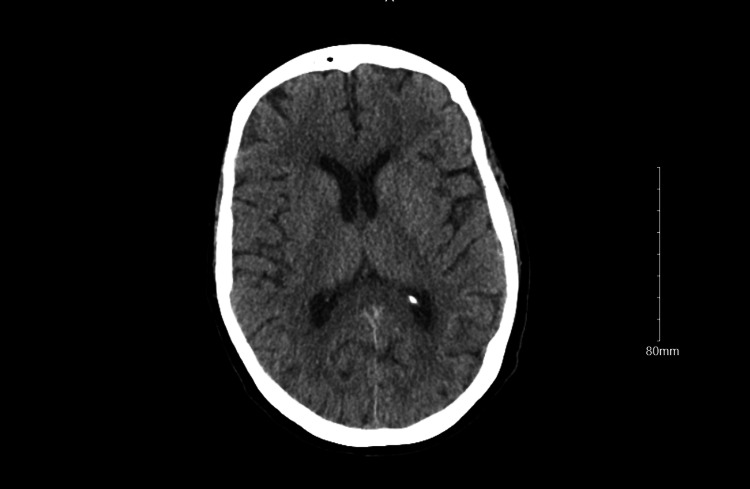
CT brain without contrast No acute intracranial abnormality.
